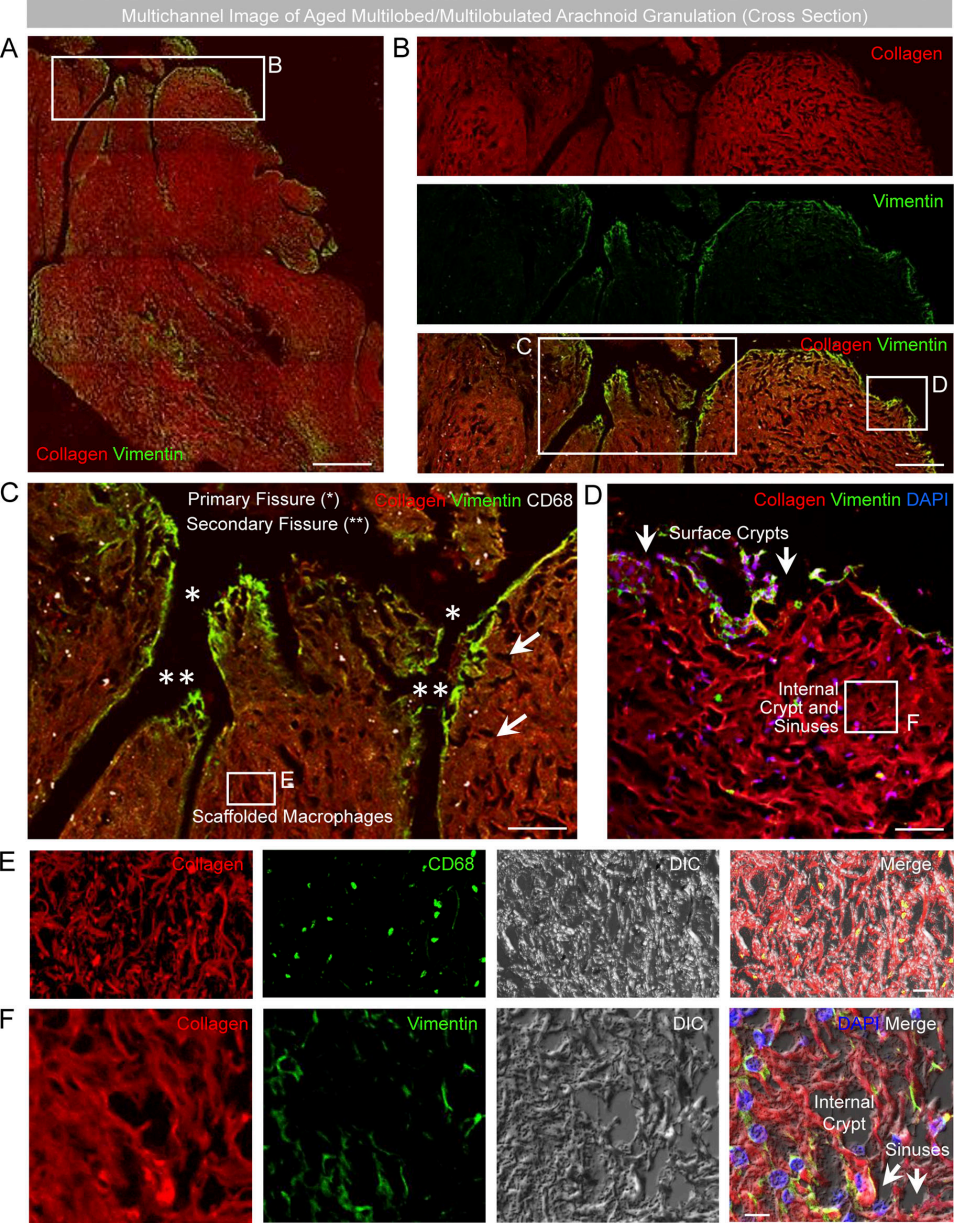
Fluorescent images of a multilobed AG depict relationships of fissures, sinuses, and crypts within the irregular stromal cavernous space network. (A) Multichannel images of a multilobed AG, shown in cross section, demonstrate its irregular morphology. (B) Cropped images of one edge show multiple indentations at the capsular surface. (C) The larger indentations with vimentin-positive lining delineate meningothelium-coated fissures, which impart distinct lobes. (D) Smaller surface apertures represent sinuses and crypt openings that are shown in communication with the periapical space. (E and F) Internal CD68^+^ macrophages (C and E) and sinuses and crypts (F) are depicted in multichannel images. (A–F) Green/FITC, vimentin or CD68; red/CY3, pan-collagen; white/CY5, CD68; blue, DAPI; DIC, differential interference contrast. Scale bars: (A) 100 µm; (B) 50 µm; (C) 25 µm; (D–F) 10 µm. Single asterisks (*) represent primary fissures. Double asterisks () represent secondary fissures that branch off of primary fissures. Arrows represent sinus and crypt spaces. Images are confocal z-stacks. Note that internal cavernous spaces are formed by smaller sinuses and larger, lake-like crypts that communicate directly with the periapical region at multiple foci of surface capsular deficiency. Data are representative of multilobed morphology from eight old decedents, studied on >75 independent experiments.
